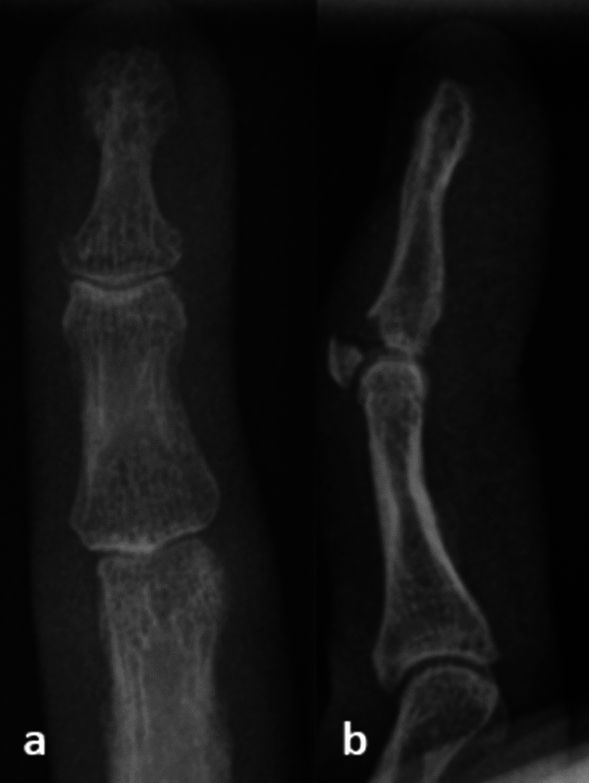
X-ray of a bony mallet finger: a posterior–anterior and b lateral
